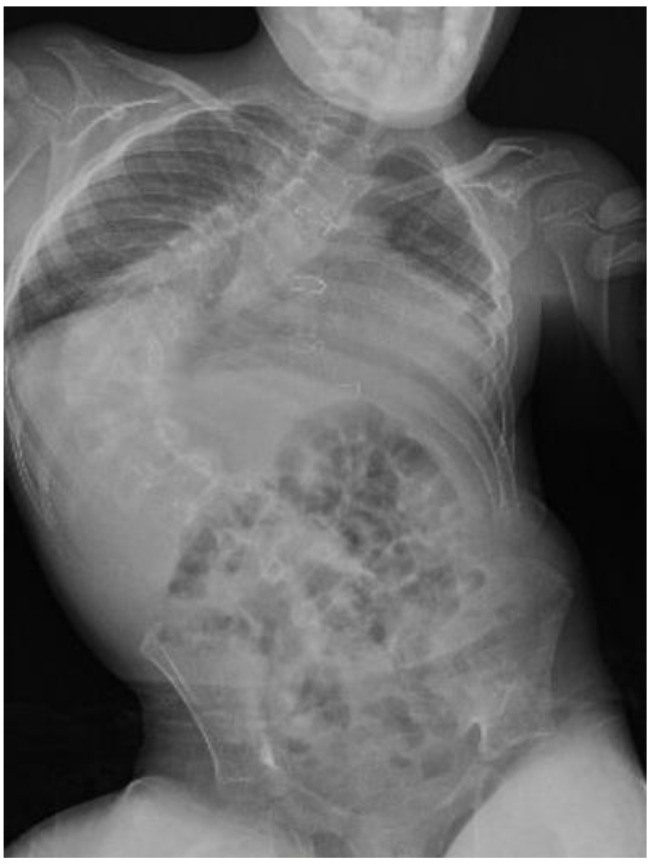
X-ray before surgery with progressive scoliosis, Cobb angle 95°.
